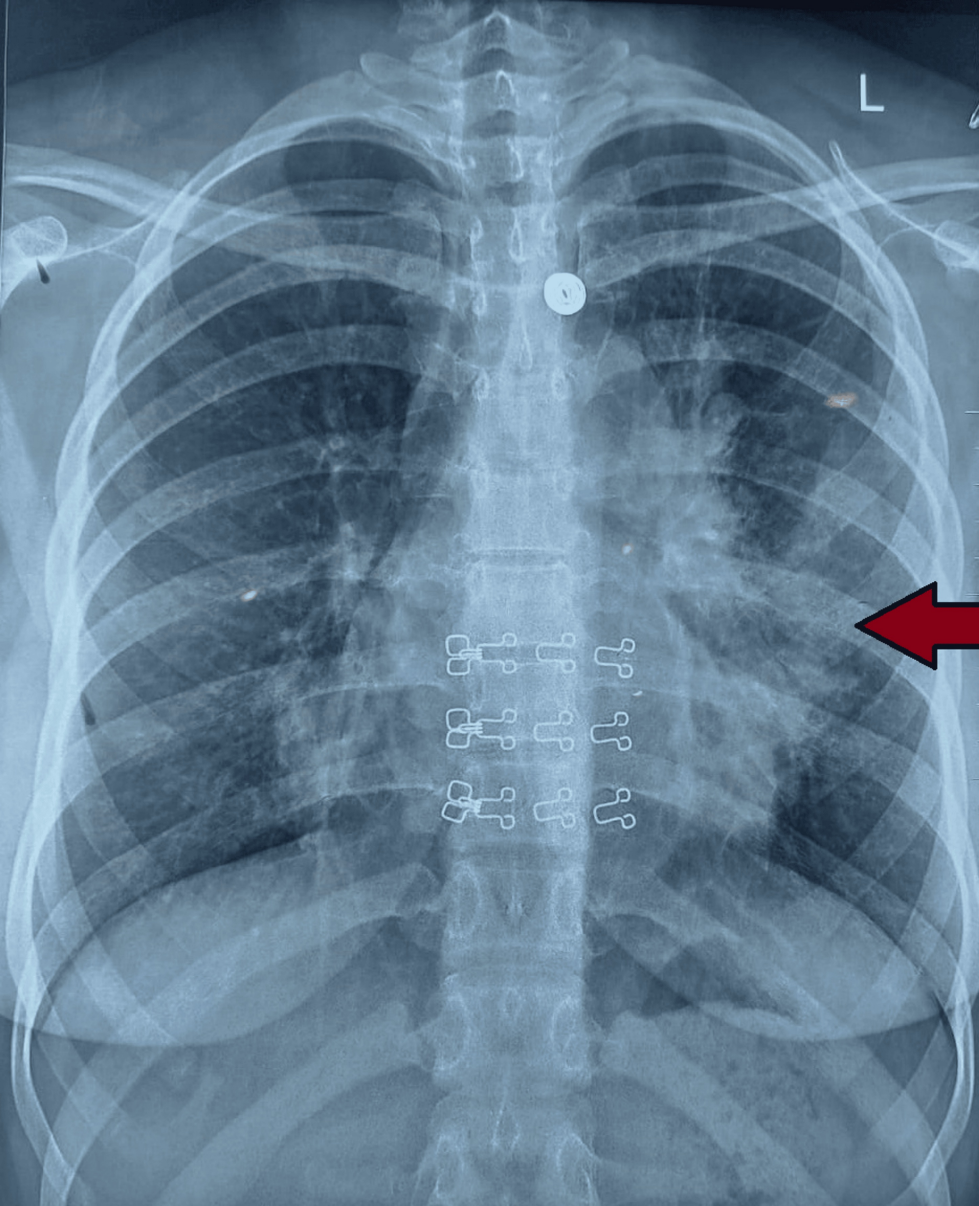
Chest X-ray showing left-sided consolidatory changes
